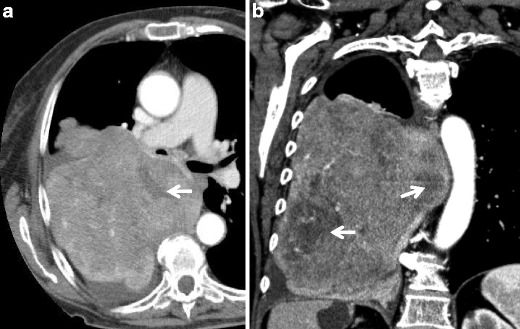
Unusual case of giant solitary fibrous tumour; contrast-enhanced CT, axial (a) and coronal plane (b) showing a bulky and inhomogeneous contrast-enhancing mass of the right hemithorax. The mediastinal structures are compressed and contralaterally migrated. The hypodense areas (*white arrows*) may represent the presence of necrosis, haemorrhage or myxoid tissue
